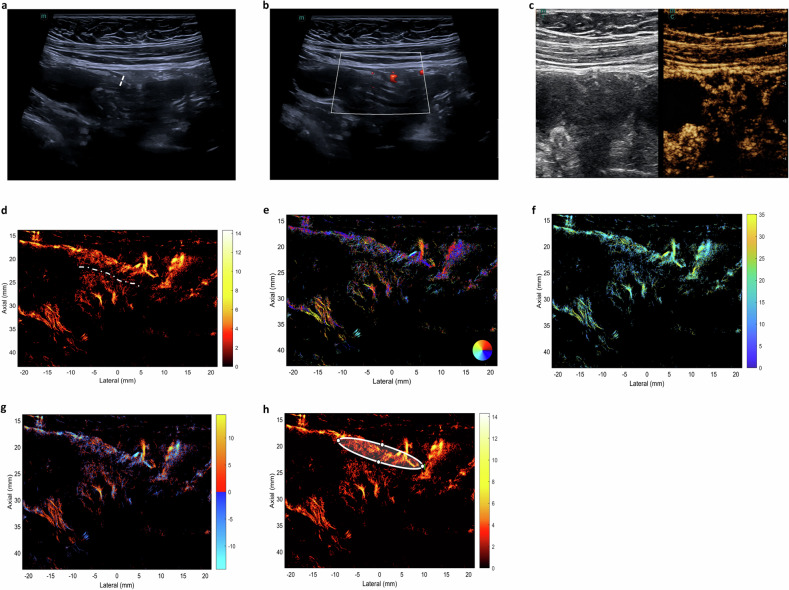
A 41-year-old female patient diagnosed with inactive Crohn’s disease (CD). The value of total simple endoscopy score for CD (SES-CD) is 1. On longitudinal scan, the bowel wall layers are clear. The bowel wall thickness of the ileum is 3.2 mm (white dashed line, a); color Doppler signal of the inflamed bowel wall displays dot-like blood flow signals (Limberg II, b); contrast-enhanced ultrasound (CEUS) of the thickest bowel wall displays enhancement of submucosa (pattern III, c); super-resolution CEUS (SR-CEUS) vascular density map of the inflamed bowel wall shows fine branching vessels in the inflamed bowel wall. The white dashed line demarcates the intestinal lumen (d). SR-CEUS vascular density direction map of the inflamed bowel wall is shown (e), SR-CEUS flow velocity map of the inflamed bowel wall is shown (f), SR-CEUS flow direction map of the inflamed bowel wall is shown (g). By SR-CEUS offline analyzing, quantitative parameters were automatically derived from a manually placed region of interest (ROI) over the inflamed bowel wall (h)
